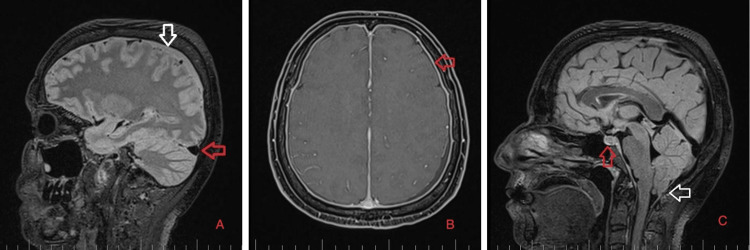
Brain MRI on February 1, 2023. A: Sagittal T2-weighted images. The white arrow shows subdural collection up to 5 mm in the parietal area, and the red arrow shows the distended convex appearance of the transverse sinus (signs of venous distension). B: Axial T1 images with contrast. The red arrow shows diffuse, smooth dural thickening and enhancement. C: Sagittal T2-weighted images. The red arrow shows slight inhomogeneity and pituitary gland enlargement, and the white arrow shows cerebellar tonsil herniation into the foramen magnum.
